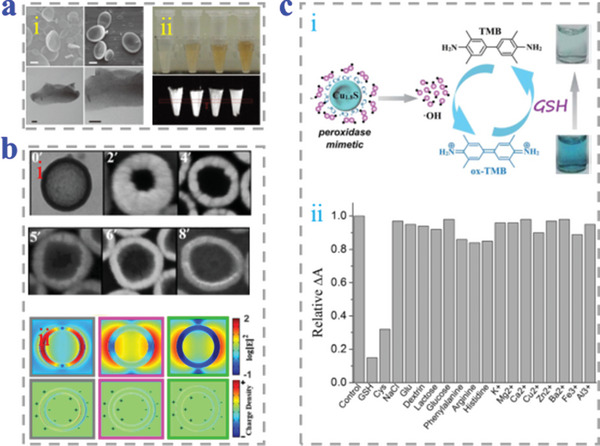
ELPs for detection and imaging. a) ELMs with performance in MRI. a‐i) SEM images and TEM images of ELMs. a‐ii) Performance of ELPs at different concentrations in MRI. Reproduced with permission.^[^
